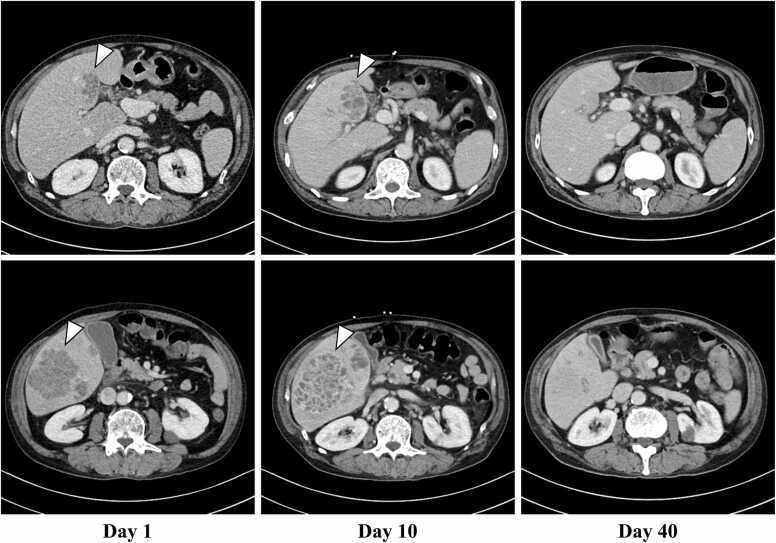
Enhanced Computed tomography scan series of liver abscess. There is multifocal liver abscess (white arrowhead) in S4, S5–6 of the liver on Day 1 and 10. Liver abscess appears to have resolved on day 40.
